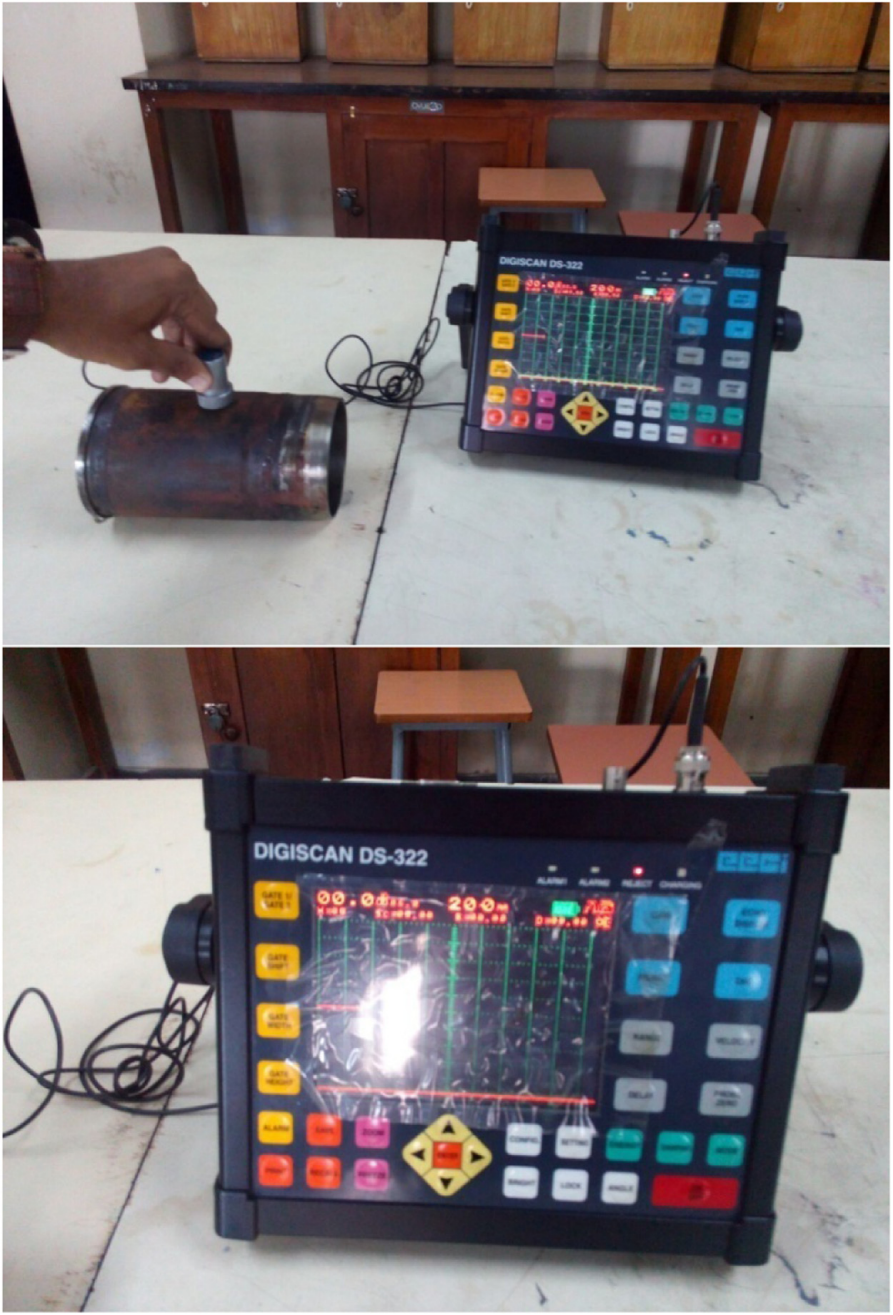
Ultrasonic inspection test.
